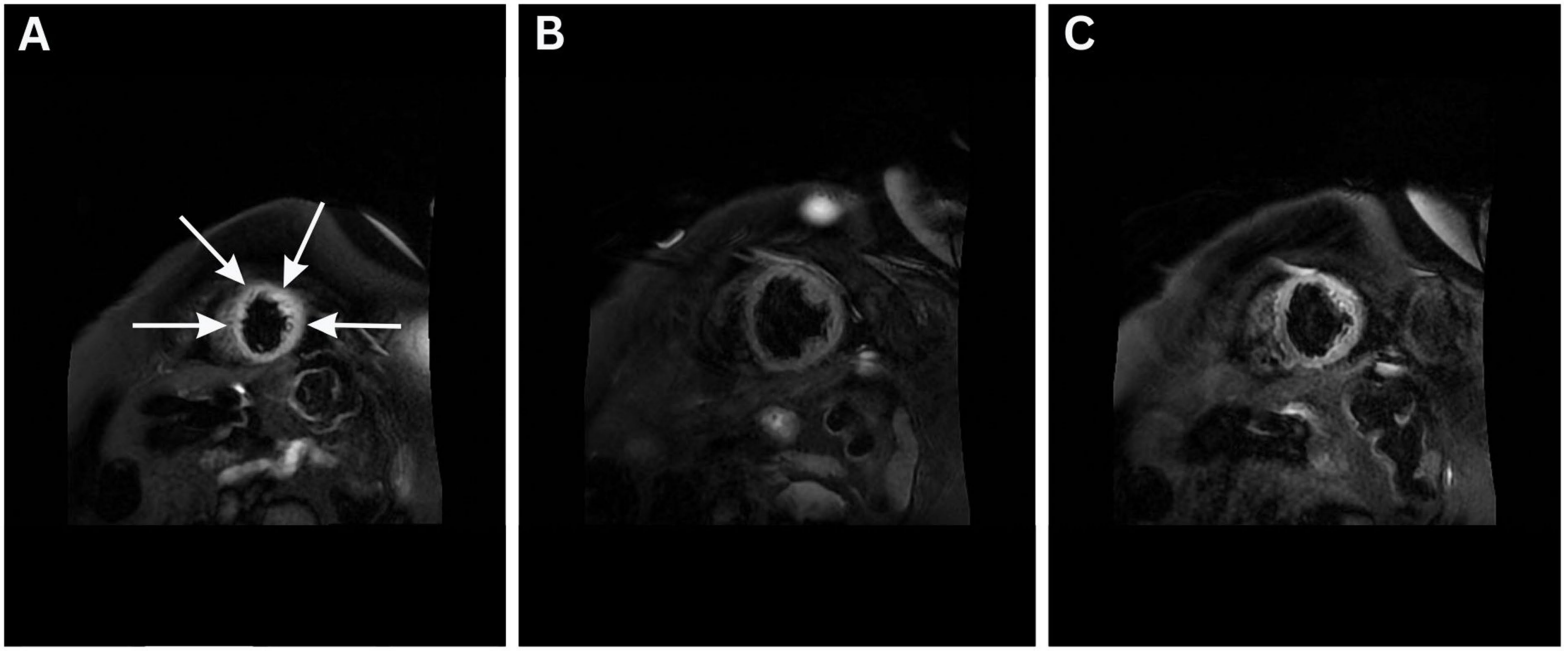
Cardiac magnetic resonance imaging performed on the 6th day of admission (A–C), T2 STIR images obtained showing apical and mid-ventricular edema (arrows in A) and akinesia with severely reduced left ventricular ejection fraction (LVEF-3D: 22%). Volumetric analysis revealed normal left ventricular size (LVEDV/BSA = 80 mL/m^2^) and no evidence of left ventricular hypertrophy. These findings are consistent with Takotsubo cardiomyopathy and help exclude infarction and myocarditis as differential diagnoses.
